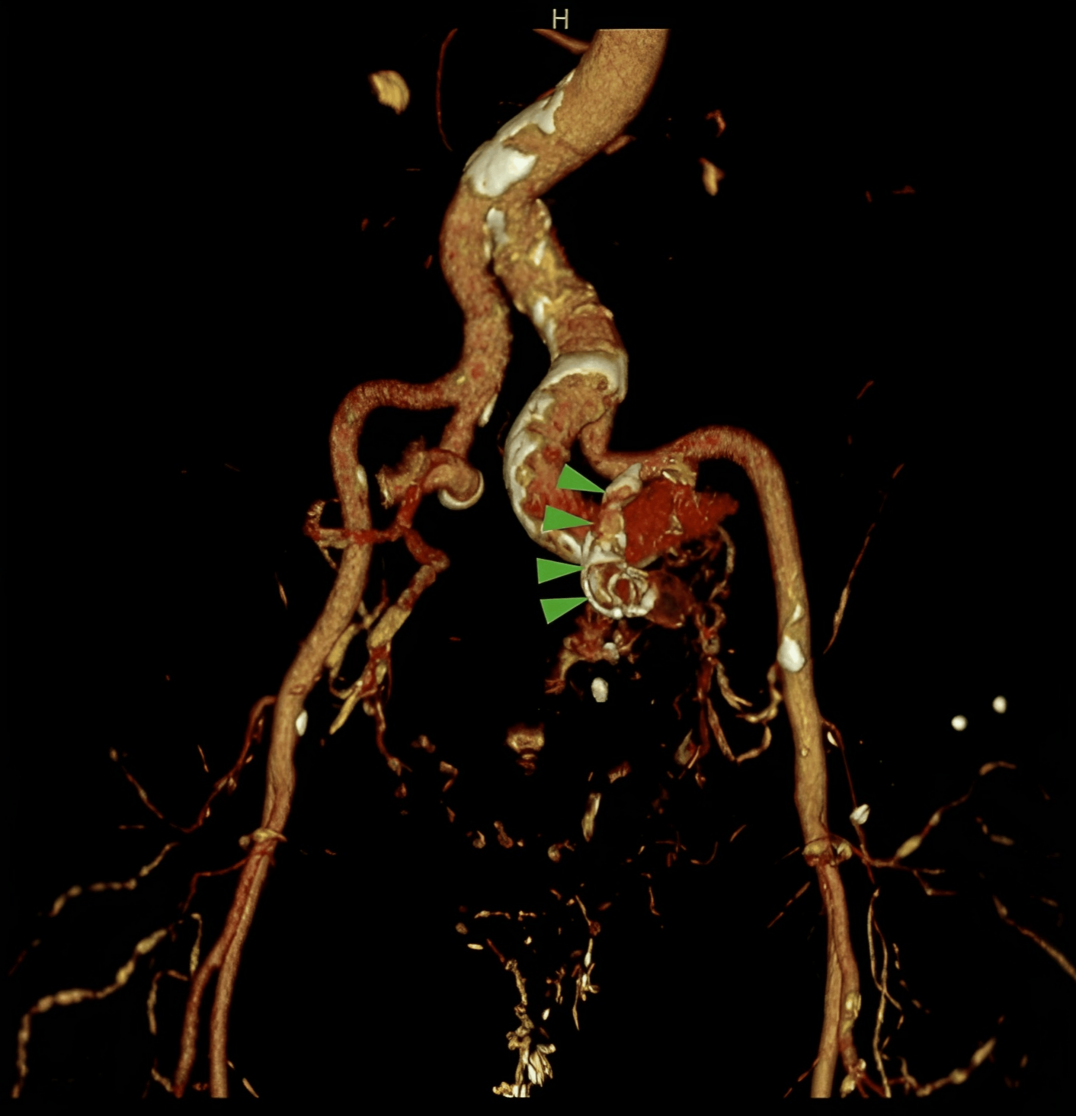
Three-dimensional CTA reconstruction demonstrates no right common iliac vein filling and thrombosis of a long segment of the right IIA proximal to the AVF plug. The location of the plug is demonstrated with green arrows. CTA: Computed tomography angiography. The 3D reconstruction using a Siemens Syndo, via the workstation at the Veterans Association (VA). All VA products are licensed (by paying a fee), and the reconstruction was done by Dr. Radu Serban.
